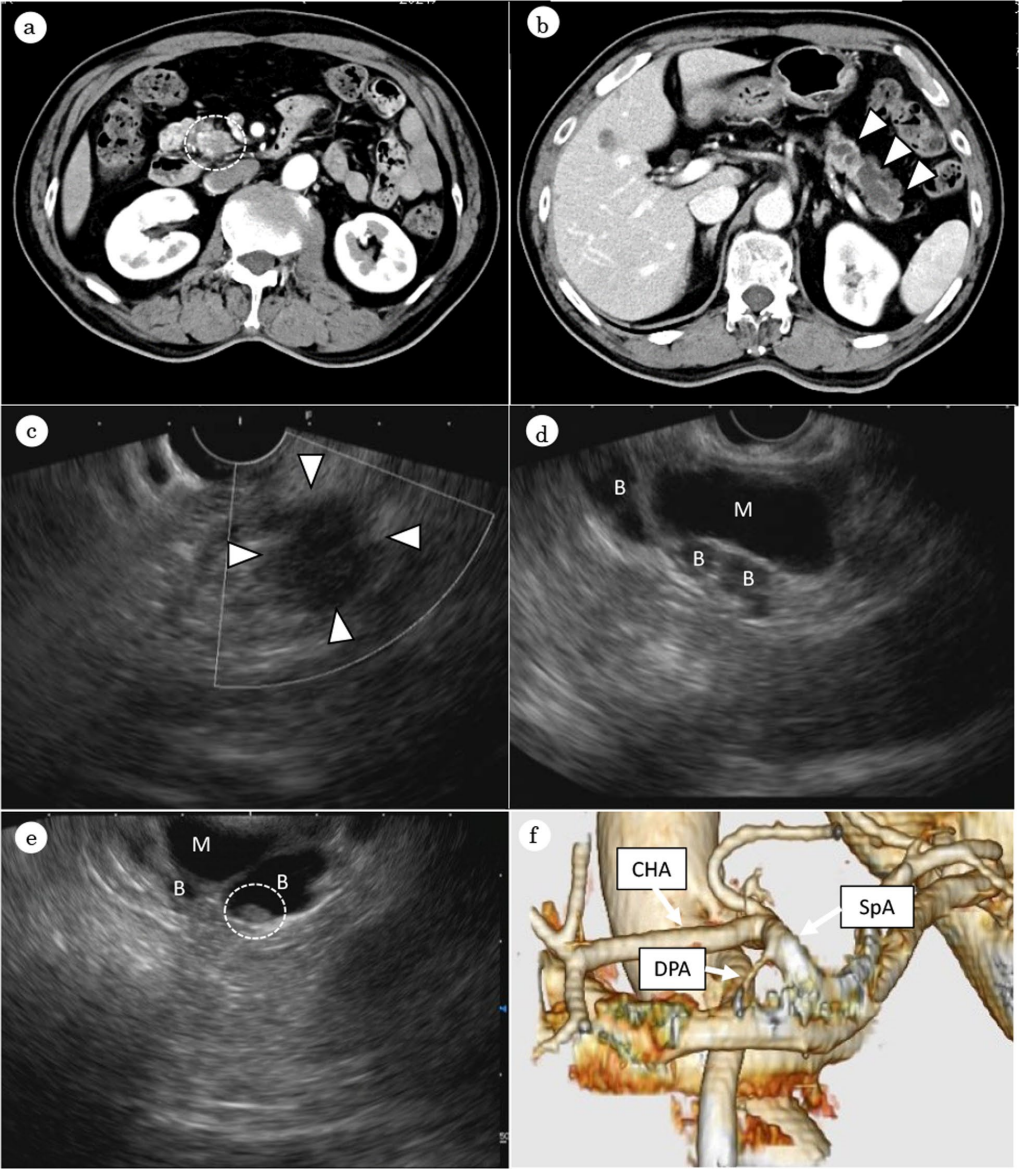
Contrast-enhanced CT revealed a hypovascular mass in the uncus of the pancreas, suspected of pancreatic cancer (encircled by a white dotted line) (a). Main pancreatic duct was dilated, 13 mm in size and multiple cystic lesions were also seen, indicating mixed-type IPMN (arrowhead) (b). Endoscopic ultrasonography revealed a hypoechoic mass in the uncus of the pancreas (arrowhead) (c) and dilatation of the main (M) and branch (B) pancreatic ducts (d) with mural nodule, 4.6 mm in size (encircled by a white dotted line) (e). Preoperative 3D-CT. The DPA originated from the proximal SpA (f). *IPMN* intraductal papillary mucinous neoplasm, *DPA* dorsal pancreatic artery, *SpA* splenic artery, *CHA* common hepatic artery
